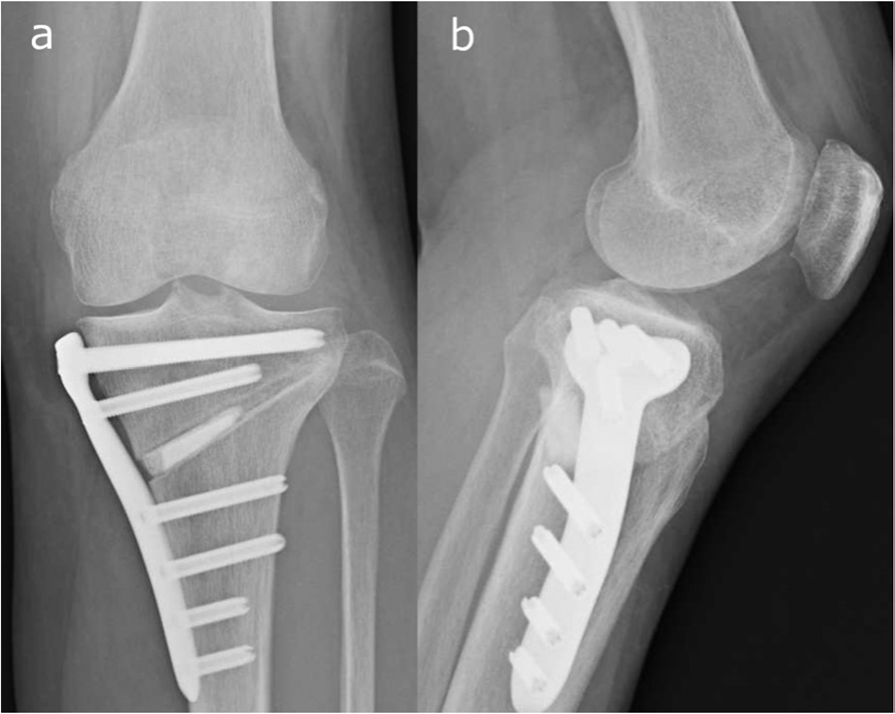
Radiographs after medial open-wedge HTO. A Anteroposterior radiograph. B Lateral radiograph
